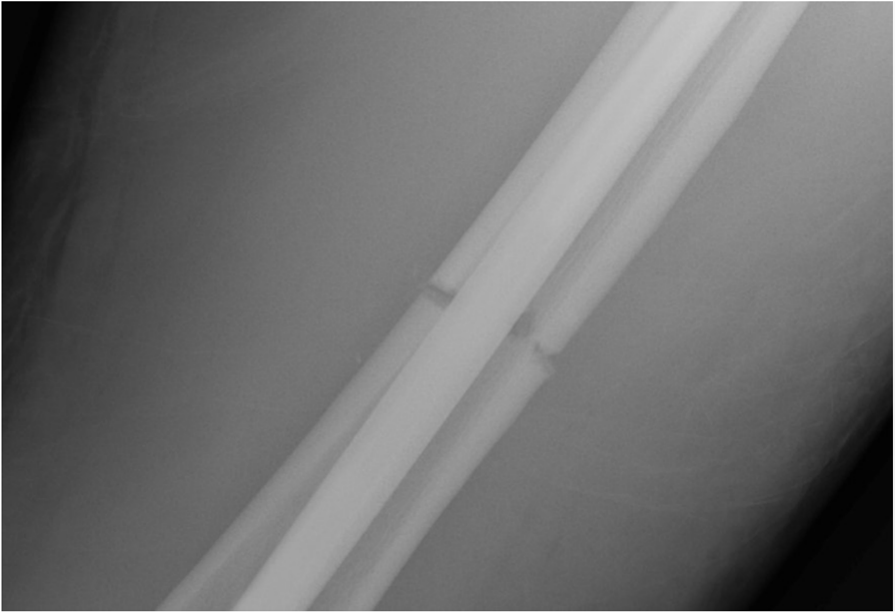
Radiographic example for CN difference > 2 mm
